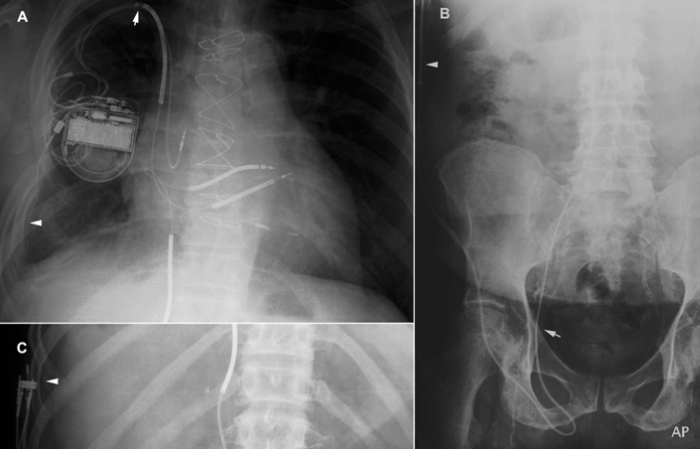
A: A posteroanterior Chest X-ray showing the ICD generator placed in right prepectoral region. Note the new ileofemoral RV defibrillation lead and the preexisting chronic RV defibrillation lead in the RV. Arrow pointing upwards shows the subclavian crush. Arrowhead shows the RV coil with the 25 cm extension in the subcutaneous tunnel. Figure B shows the new RV lead in the right iliac vein, making a U turn in the groin and traversing upward to the chest through the subcutaneous tunnel created. Figure C shows the RV coil in the subcutaneous tunnel and the Y-adaptor extension used to increase the length of the RV lead to reach the device. Note the RV pacing/sensing component and the IVC coil of the lead is capped.
